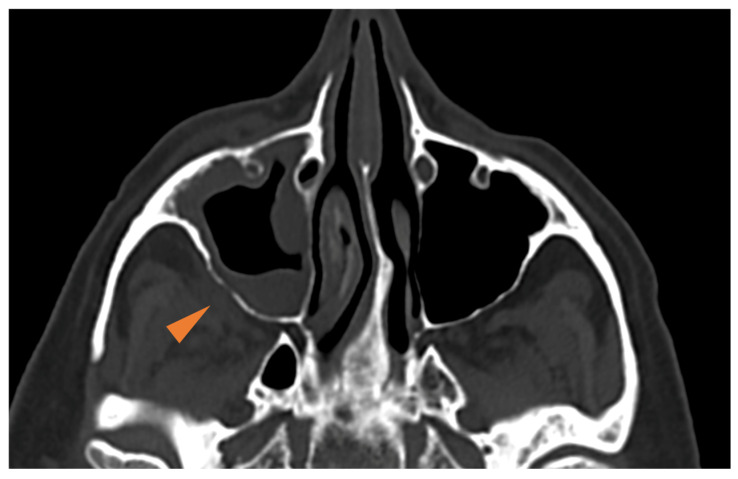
54-year-old male confirmed with right AIFR (*Mucor*). CT axial section showing right periantral fat infiltration (arrowhead) associated with subtle thinning of the posterior maxillary bony wall. See Figure 1C.
